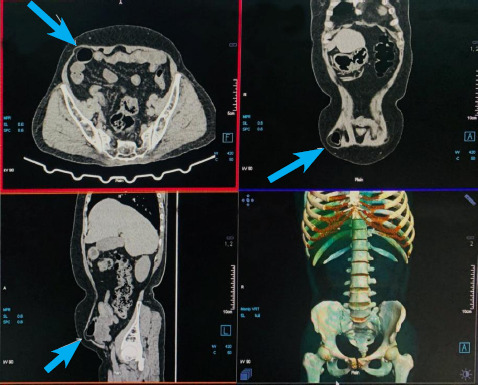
CT of the patient (arrow showing Spigelian hernia).
